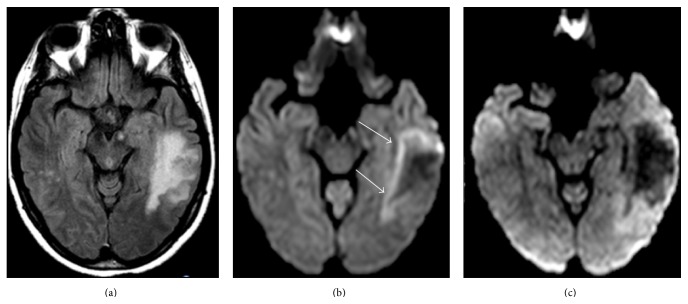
(a) T2 FLAIR and (b) DWI images demonstrate a large PML lesion. (b) DWI demonstrates cytotoxic edema along the advancing edge of the lesion (arrows) surrounding the quiescent center. (c) Repeat MR DWI image following PLEX demonstrates absence of the hyperintense rim suggesting that disease progression has resolved.
